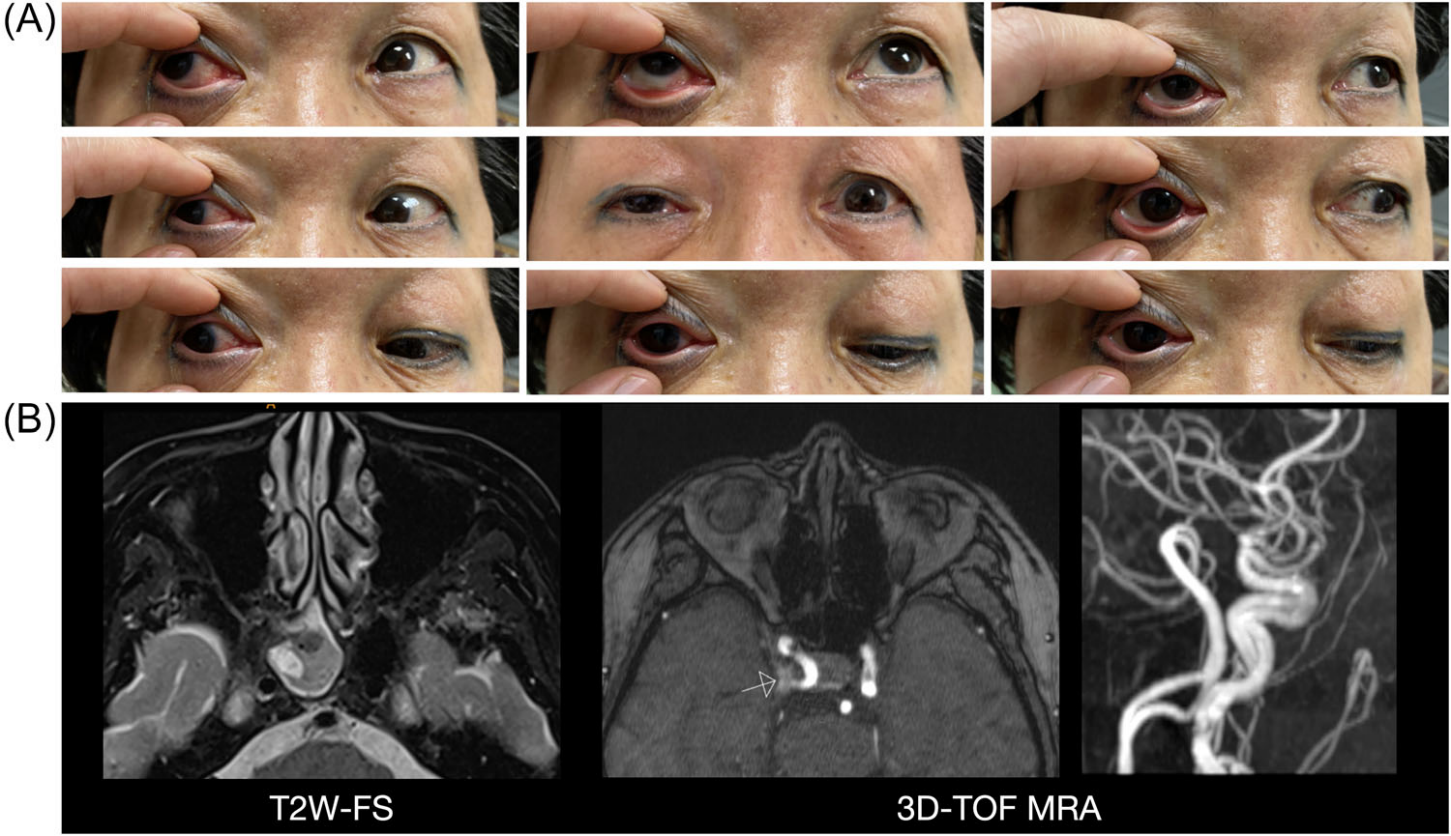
Initial presentation: right ptosis and limited eye movements (A). T2‐weighted fat‐saturated magnetic resonance imaging (T2W‐FS MRI) shows left sphenoid sinusitis (left). Three‐dimensional time‐of‐flight magnetic resonance angiography (3D‐TOF MRA) reveals focal signal changes near the right cavernous sinus (arrow, right) (B).
